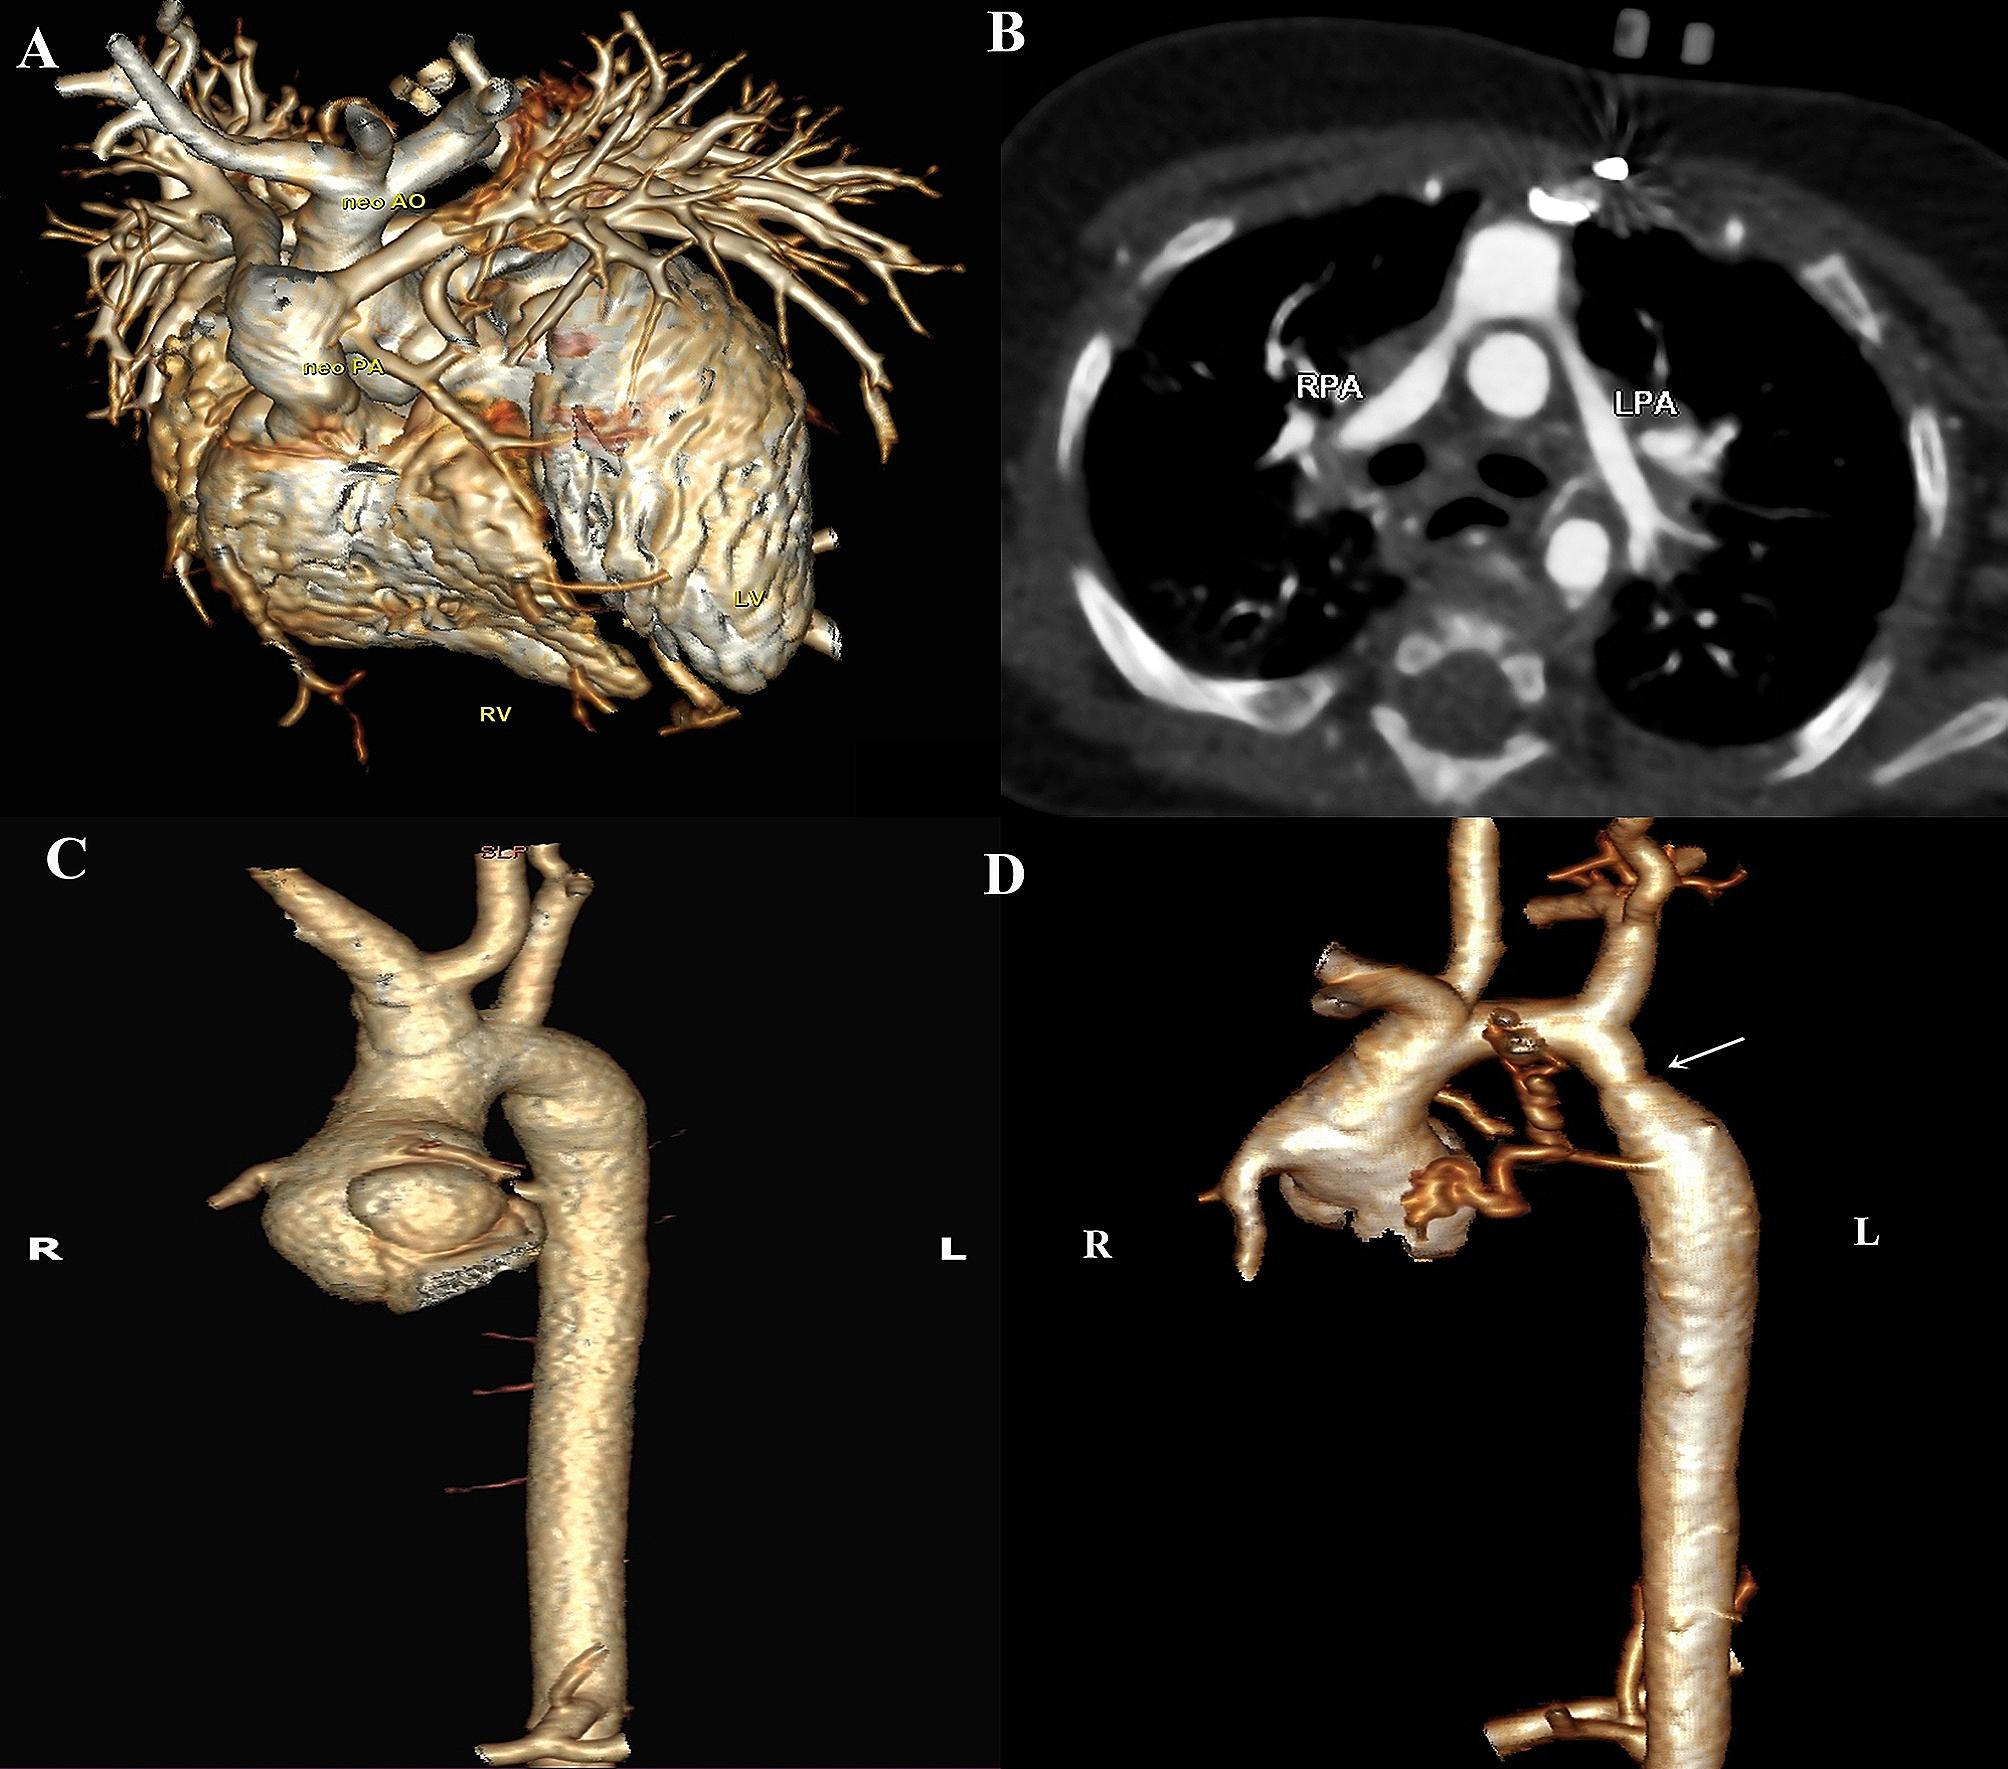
Abnormalities detected in post-ASO MSCT of some of the included cases. (A) Volume-rendered CT image showing neo-PA with its two branches draping around the neo-aorta. (B) Axial oblique CT image showing stenosis of both RPA and LPA. (C) Volume-rendered CT image of the aorta showing dilated neo-aortic root and STJ. Note the common origin of brachiocephalic and left common carotid arteries (Brachiocephalic trunk). high takeoff of both coronary arteries. (D) Volume-rendered CT images of the heart and aorta showing aortic coarctation and major aortopulmonary collateral arteries; note the high takeoff of both coronary arteries. LPA: left pulmonary artery, neo-PA: neo-pulmonary artery, neo-Ao: neo-aorta, RPA: right pulmonary artery
